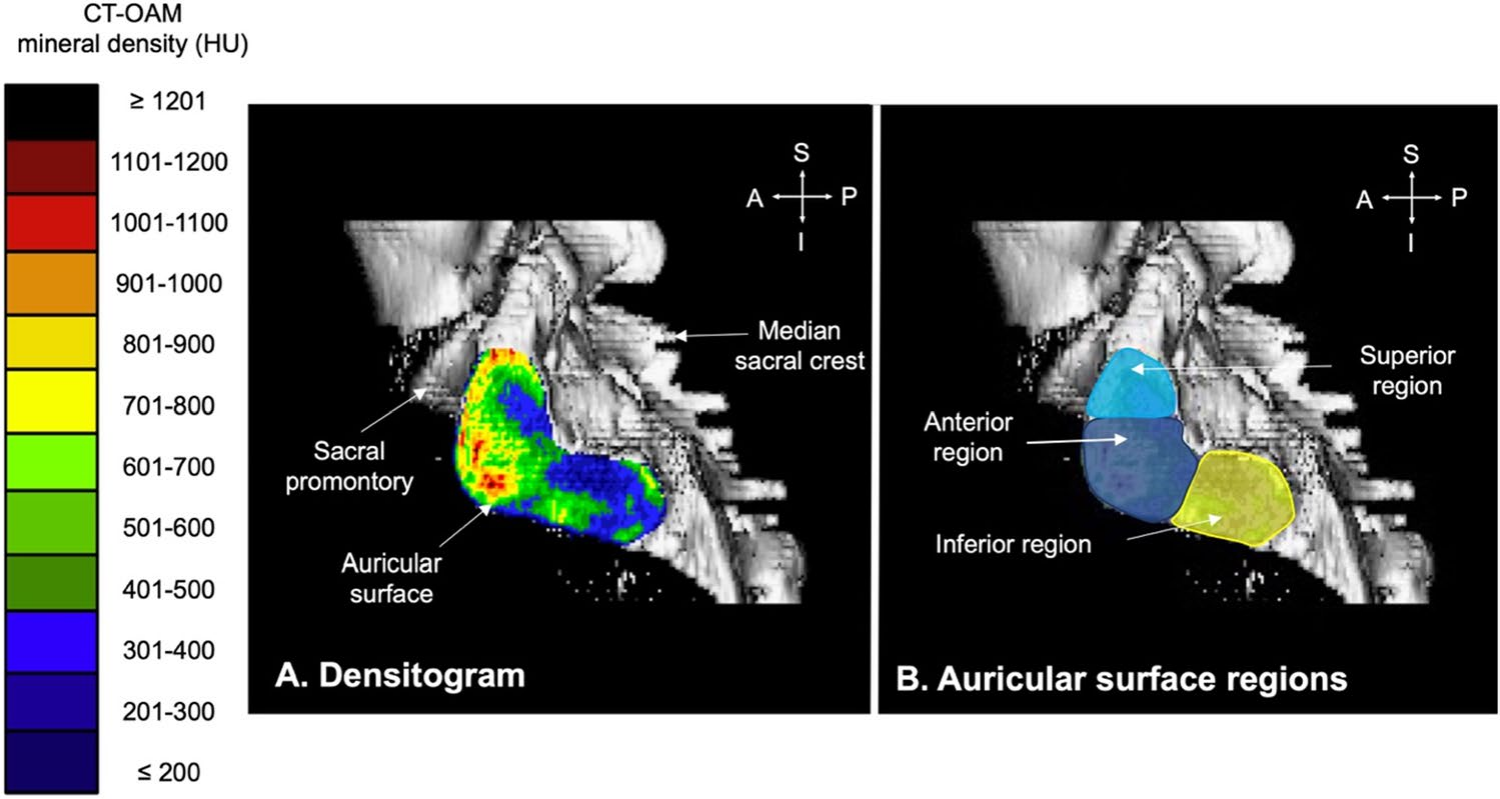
(A) Example of a sacral auricular surface densitogram with the Hounsfield unit (HU) scale on the left. (B) the same densitogram divided into the three regions, light blue: superior region, dark blue: anterior region and yellow: inferior region. The area of each region calculated the mean Hounsfield units’ value of that region. *CT-OAM* computed tomography osteoabsorptiometry, *A* anterior, *I* inferior, *P* posterior, *S* superior.
